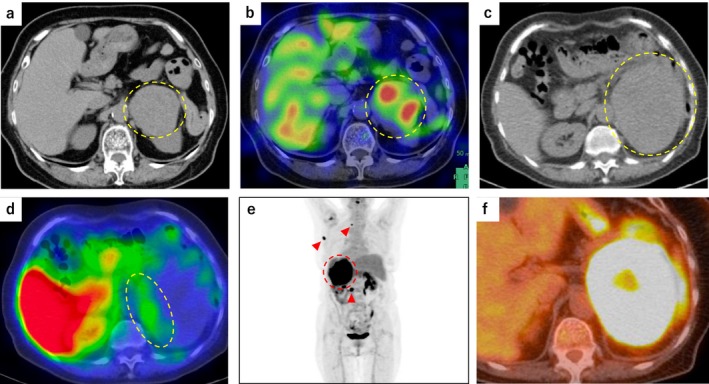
MIBG scintigraphy and FDG‐PET/CT findings. (a, b) At diagnosis, a non‐contrast CT image (a) at the same axial level as the MIBG scintigraphy image (b) shows a left adrenal mass with MIBG uptake (yellow dotted circle). (c, d) Four months later, a non‐contrast CT image (c) corresponding to the MIBG scintigraphy slice (d) shows the size of the left adrenal mass, while MIBG uptake is decreased but partially retained (yellow dotted circle). (e) FDG‐PET/CT maximum intensity projection (MIP) image shows intense FDG uptake in the left adrenal mass (red dotted circle) and newly developed lymphadenopathy in the para‐aortic, left axillary, and left supraclavicular regions (red arrowheads). (f) Axial FDG‐PET/CT image demonstrates intense FDG uptake in the left adrenal mass.
